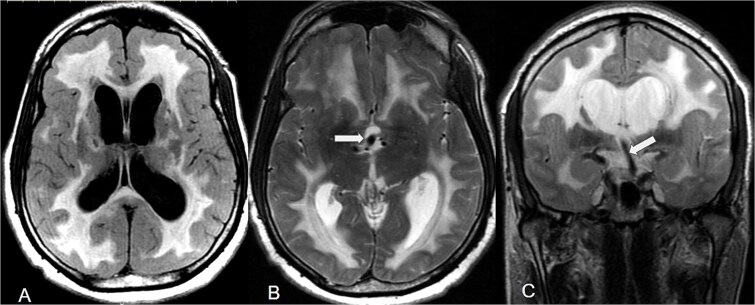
MRI of the brain demonstrating obstructive hydrocephalus secondary to vertebrobasilar dolichoectasia. (A) Axial FLAIR image and (B) axial T2-weighted image demonstrating dilatation of the lateral and third ventricles with periventricular hyperintensity consistent with transependymal oedema. (C) Coronal T2-weighted image demonstrating an ectatic basilar artery compressing the floor of the third ventricle.
